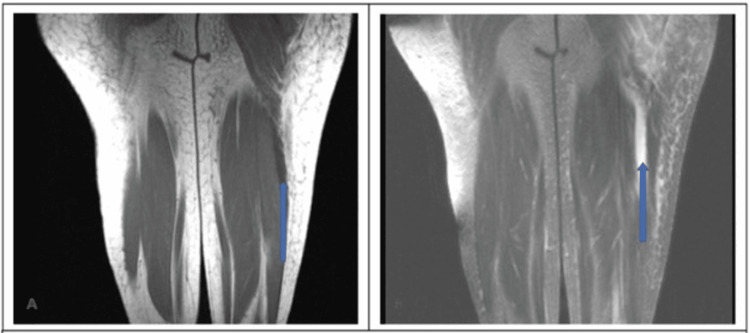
Coronal T1 (A) and T2 (B)- weighted MRI of the left lower extremity showing a large hematoma/fluid tracking in the fascial planes surrounding lateral biceps femoris muscle (blue arrows)
